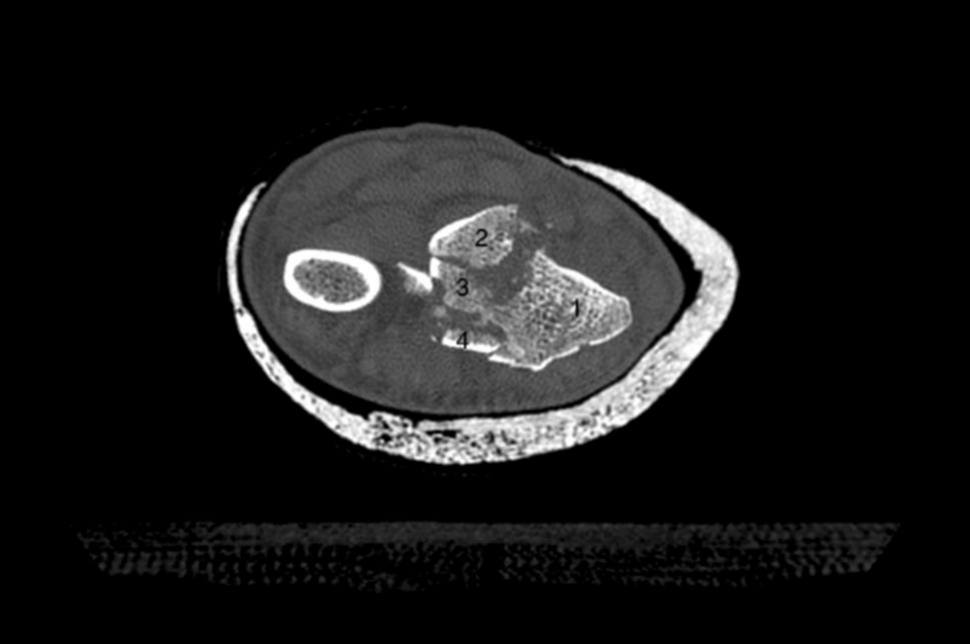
CT scan of an axial view with the numbered intraarticular fragments 1: radial styloid, 2: dorso-ulnar fragment, 3: fragment of the intermediate column 4: volar fragment
